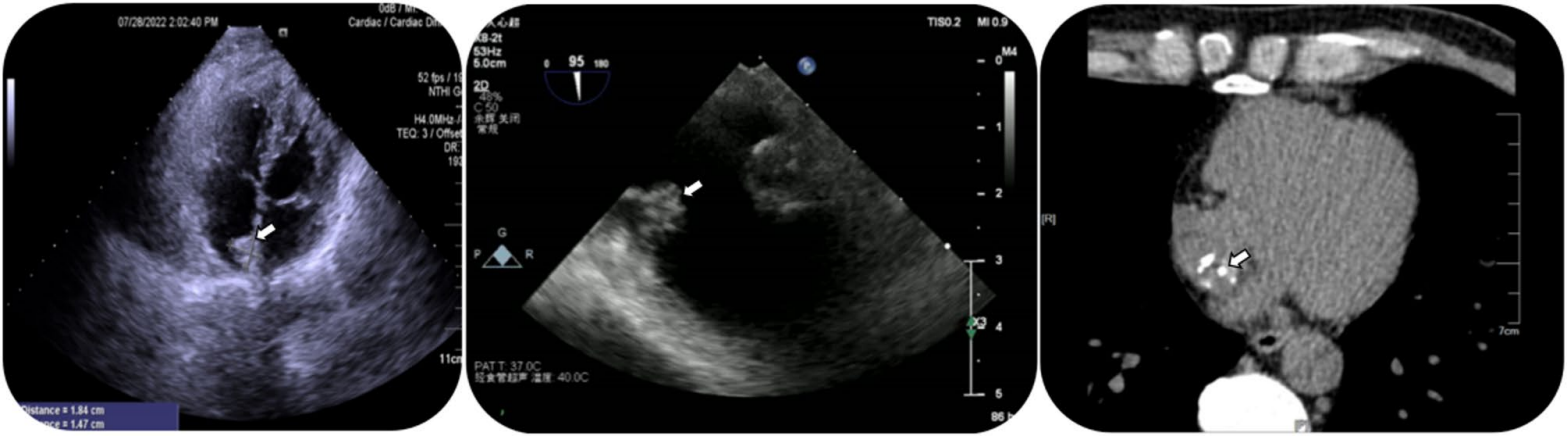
A: Two-dimensional echocardiography displays a pedunculated calcified mass in the right atrium. B: Transesophageal echocardiography demonstrates a 13.2 × 11.8 mm pedunculated mobile mass attached to the right side of the interatrial septum. C: Coronary CTA: intraatrial mass in the right atrium exhibits calcification
